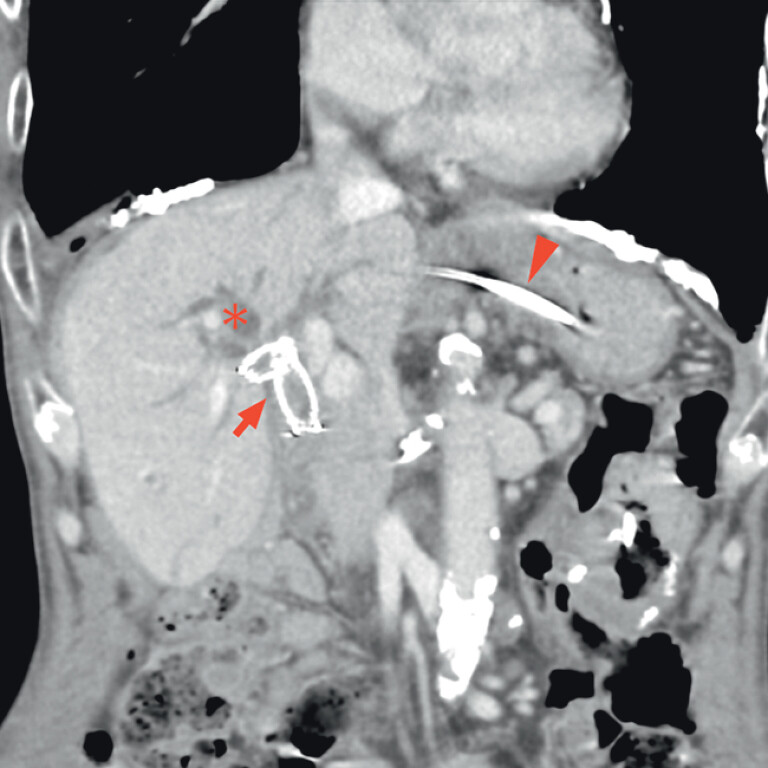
Contrast-enhanced computed tomography showing dilatation of the anterior bile duct (asterisk). For local relapse after Whipple surgery for cholangiocarcinoma, right hepatic drainage with bridging metal stent placement through the hepaticogastrostomy route had previously been performed and another metal stent placed from the jejunum to the left hepatic duct in a stent-in-stent configuration (arrow). A plastic stent had been placed across the hepaticogastrostomy route (arrowhead).
